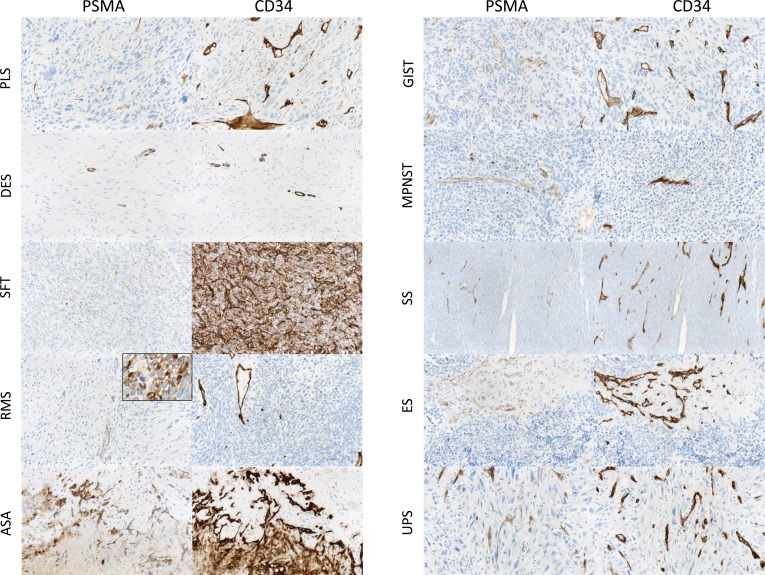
PSMA expression in the neovasculature of sarcomas Neovascular PSMA expression in different sarcoma subtypes. Vasculature was identified by means of CD34 coexpression. (PLS, pleomorphic liposarcoma; DES, desmoid-type fibromatosis; SFT, solitary fibrous tumor; RMS, rhabdomyosarcoma; ASA, angiosarcoma; GIST, gastrointestinal stromal tumor; MPNST, malignant peripheral nerve sheath tumor; SS, synovial sarcoma; ES, Ewing sarcoma; UPS, undifferentiated pleomorphic sarcoma). In one case of RMS a significant PSMA expression of tumor cells (inset) was observed.
